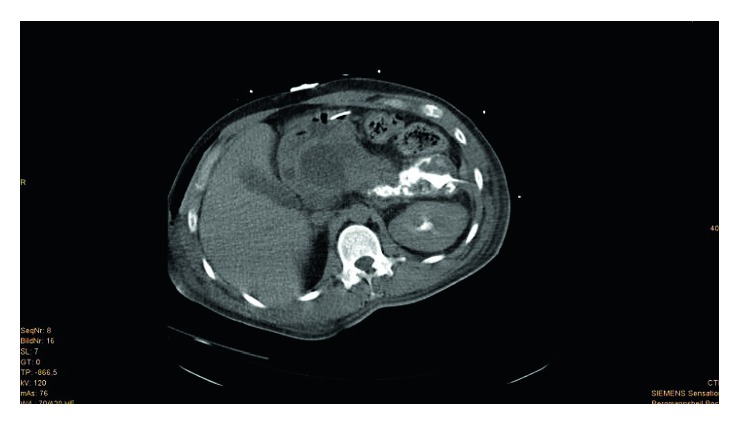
(Patient 1, scan 2) CT scan 25 days after trauma. After iatrogenic removal of drainage, a large retrogastric pseudocyst with a surrounding capsule was observed. Obviously, a narrow aisle was found where fluid accumulation continued to expand caudally below the transverse column. The pseudocyst was again punctured from the left lateral side with 9.5 French Lunderquist drainage, and contrast medium was injected into the pseudocyst cavity. There was a narrow connection path for retrogastric fluid accumulation. Thus, all the caves were drained adequately.
